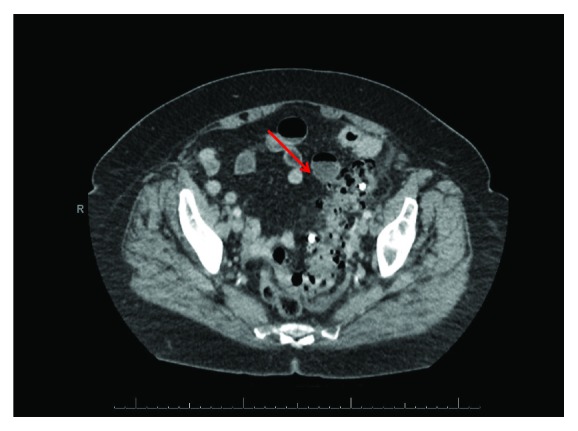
CT scan of biliary stent in sigmoid colon with diverticulosis and free air.
